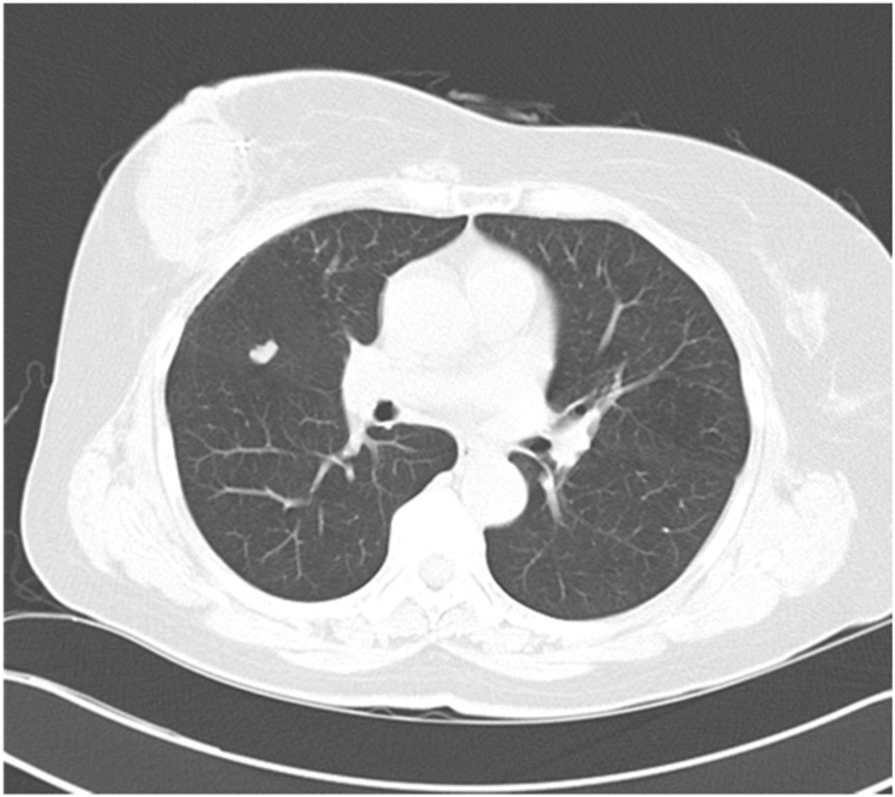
Chest CT shows a 1-cm sized, lobulating contoured nodule in the right middle lobe, attaching to the minor fissure
